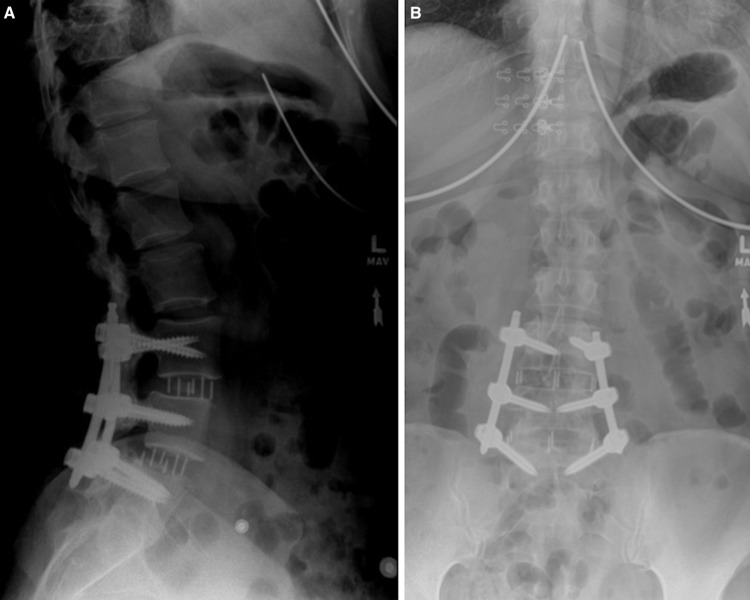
Lateral (A) and AP (B) radiograph of the lumbar spine at the six-month postoperative period.
